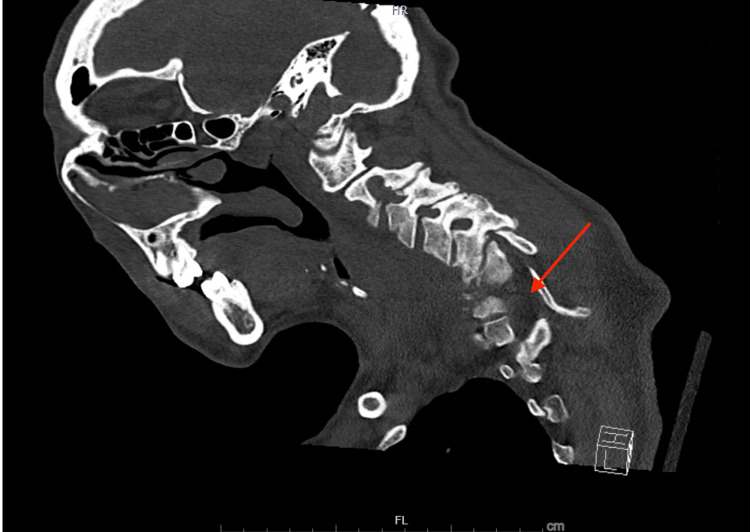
MRI of the cervical spine showing destructive changes involving C6-C7 Sagittal T2 MRI showing C6-C7 destruction with epidural phlegmon
